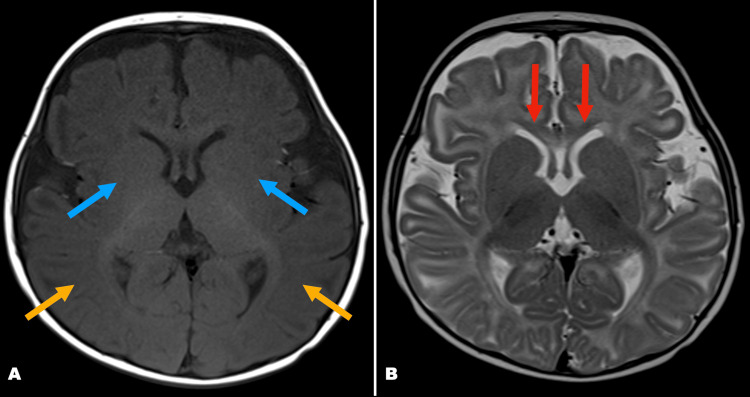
MRI findings of white matter hypomyelination Axial T1 (A) and T2 (B) images at six months demonstrating hypomyelination in parieto-occipital white matter (yellow arrows) and genu of corpus callosum (red arrows), as well as reduced conspicuity of basal ganglia (blue arrows).
